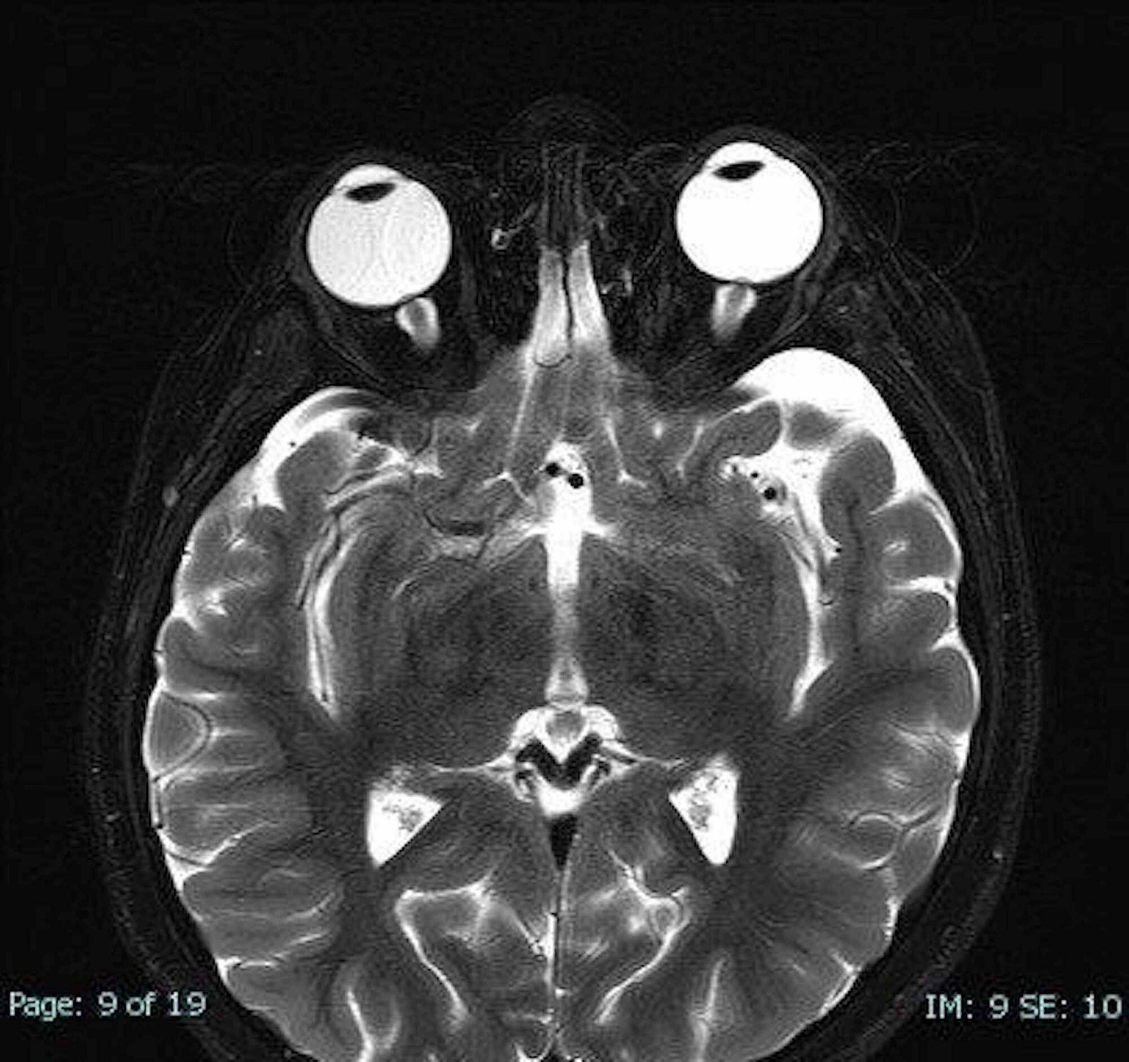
Orbital MRI exhibiting bulging of the optic nerves.
